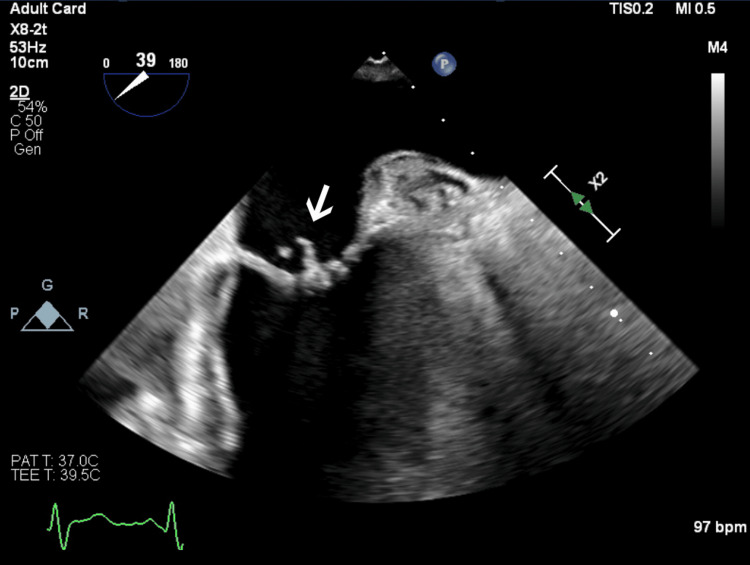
Transesophageal echocardiography image of the patient’s mitral valve clearly demonstrating posterior leaflet prolapse with a ruptured chordae tendineae.
